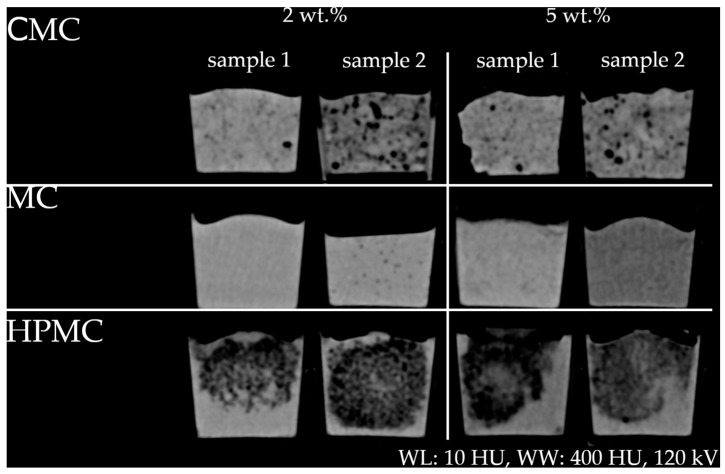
CT images of samples containing CMC, MC, and HPMC (2 wt.% and 5 wt.%) with different window settings (*U* = 120 kV). Typical broad window (WL: 10 HU, WW: 400 HU) for abdominal tissue.
